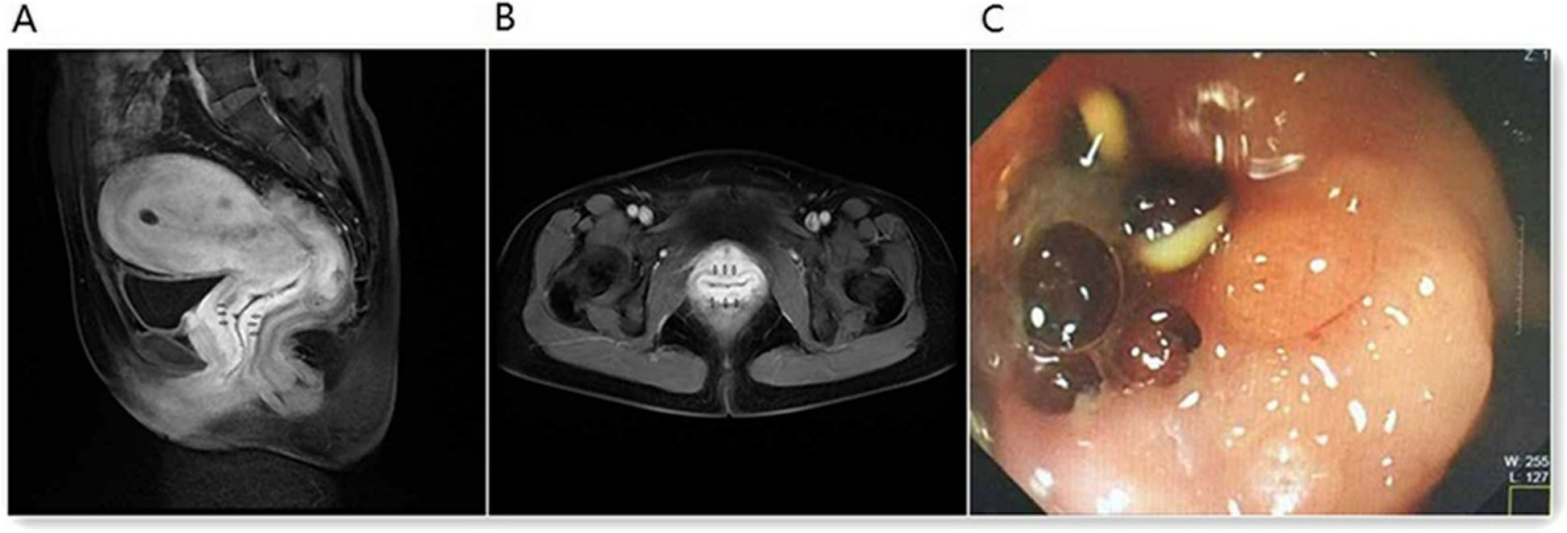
MRI and colorectal colonoscopy. a and b: Pelvic MRI showed significant thickening of the vaginal wall with enhancement. c: Colorectal colonoscopy revealed multiple lesions in the ileocecal valve and rectum
